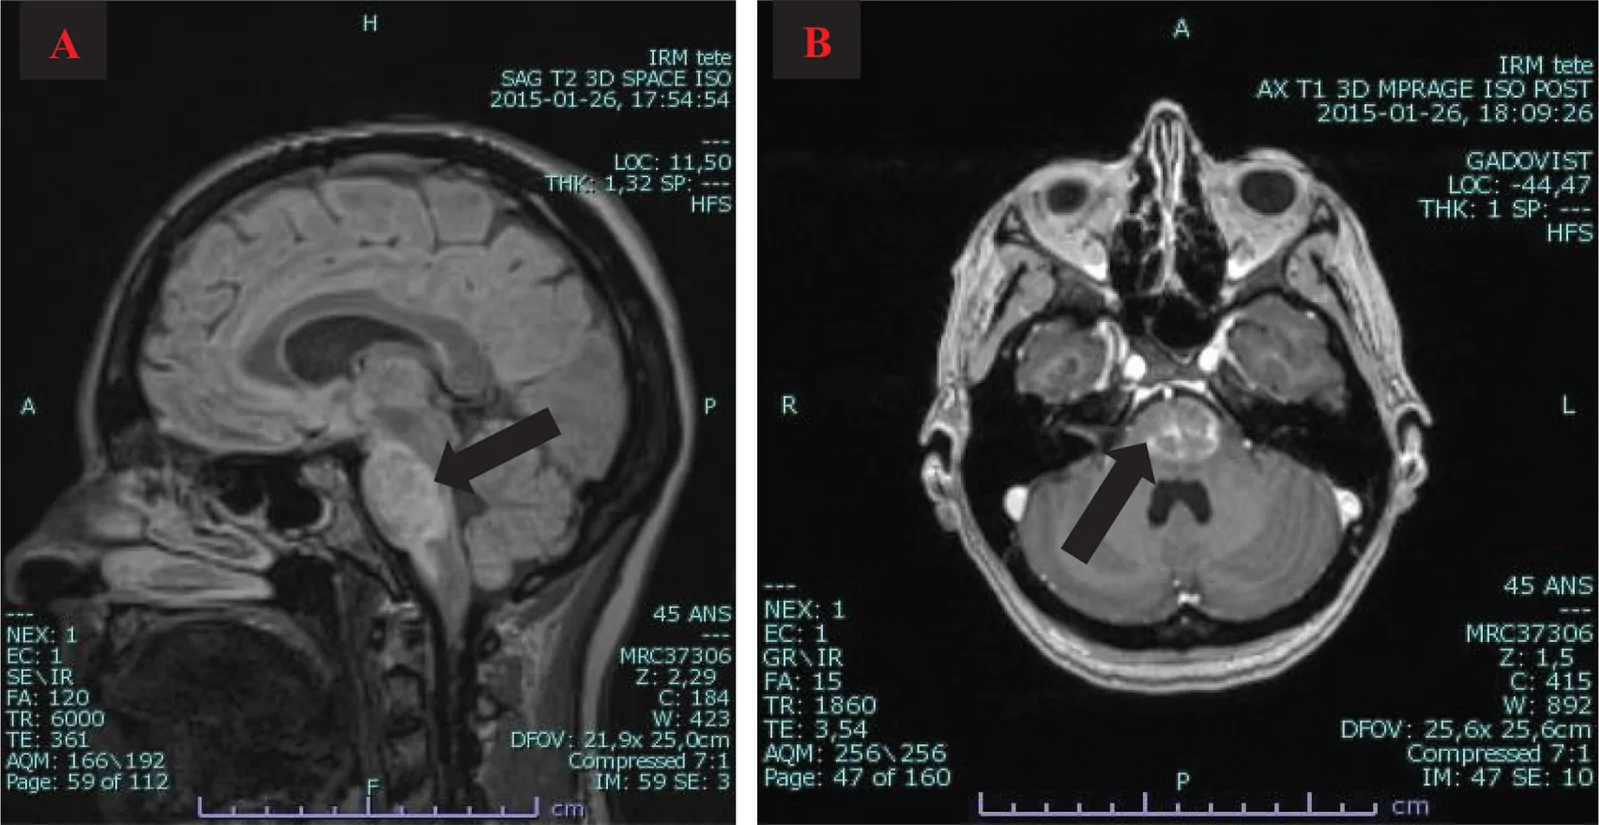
Magnetic resonance imaging (MRI) findings. (A) Sagittal MRI showing FLAIR hypersignal in pons and medulla; (B) Axial T1 MRI with gadolinium showing enhancement of the pontine lesion.
